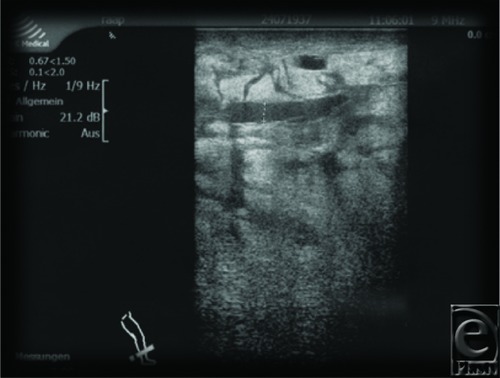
Patient 2. Preoperative sonographic control with massive enlarged lymphatics.
